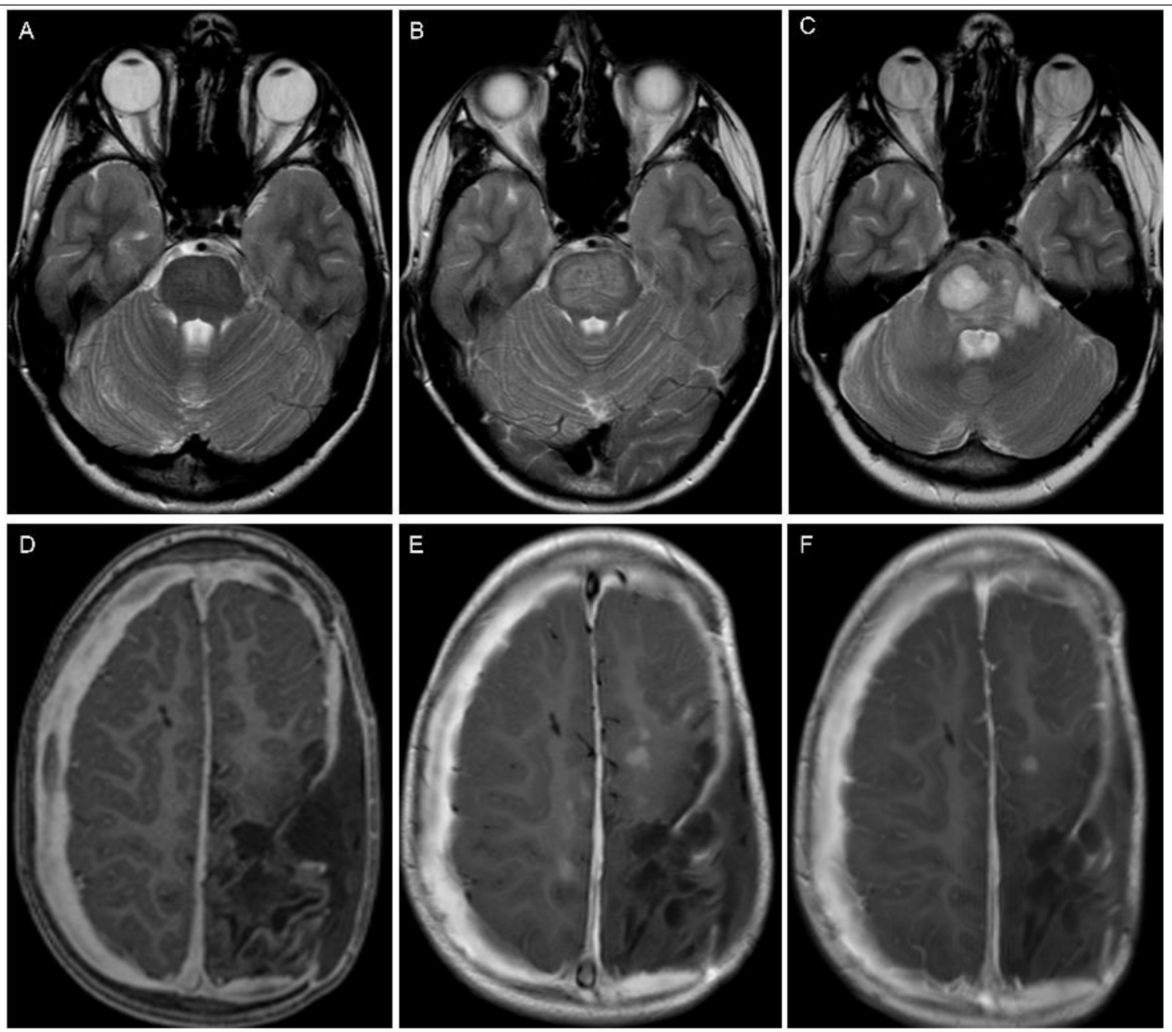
Examples of secondary lesions. (A-C) A 12-year-old boy diagnosed with a posterior fossa ependymoma developed a diffuse pontine lesion 23 months after diagnosis and a diffuse intrinsic pontine glioma was suspected. (A) Tumor manifestation started with a slight and diffuse increase in T2-signal intensity within the central pons. (B) MRI 10 months later shows the growth of the pontine T2-lesion, now fulfilling the criteria for a diffuse pontine glioma. (C) On further follow-up, the tumor is expanding into the cerebellar hemisphere. (D-F) A 2-year-old boy diagnosed with a supratentorial ependymoma (MRI at diagnosis in Fig. 1). (D) The first MRI control six weeks after proton therapy shows the left sided postoperative parenchymal defect without tumor residues but bilateral subdural hygroma on T1-weighted post-contrast images. (E) Six months after radiotherapy, new contrast-enhancing spots occur nearby the resection cavity, but also within primarily healthy brain tissue in the area of the right-sided central region. (F) Follow-up three months later shows regression of the contrast-enhancing spots, which supported the assumption of transient post-radiogenic imaging changes
